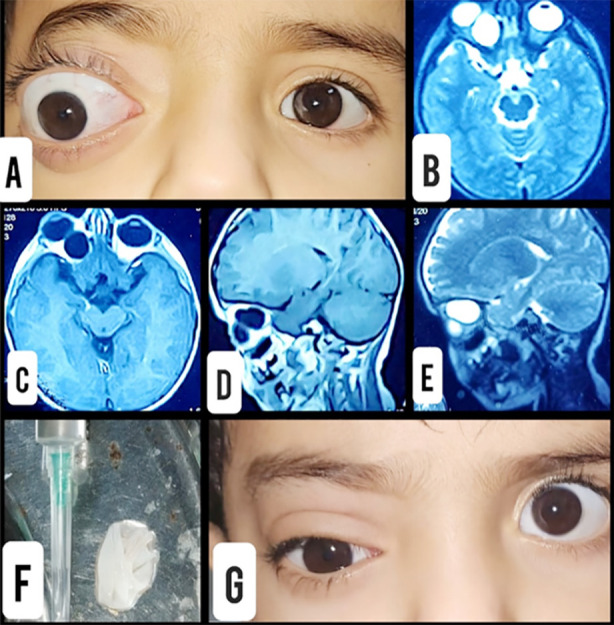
A. Right proptosis at presentation. B. T2 Axial MRI Scan. C. T1 Axial MRI Scan. D. T1 Sagittal MRI Scan. E. T2 Sagittal MRI Scan. F. Excised cyst wall with aspirated fluid. G. First Post-Operative Day.
